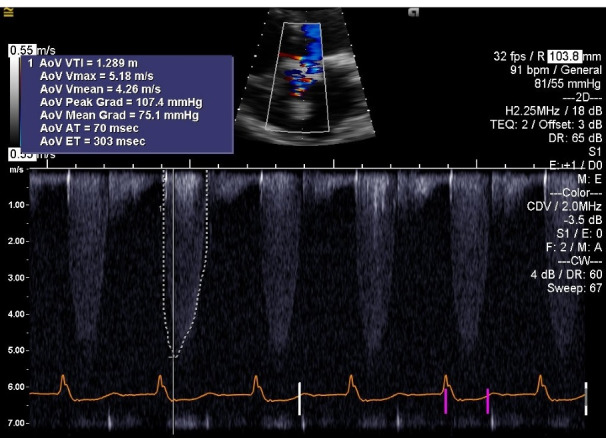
His acute decompensated heart failure was believed to be secondary to structural bio-prosthetic valve degeneration with associated critical stenosis and moderate regurgitation. Because of unstable hemodynamics, multiple comorbidities, high logistic Euro- score II of 67.0% and Society of Thoracic Surgery Risk (STS) score of 46%, the patient was deemed to be a poor surgical candidate for re-do surgical intervention. Therefore, he underwent immediate and successful TAVR with a ViV procedure with a 29 mm Medtronic Evout –R, using transfemoral approach with conscious sedation without complications. Follow up echocardiogram (Figure 2) one week post-procedure revealed prosthetic AV peak gradient of 64.3 mm Hg, mean gradient of 34.5 mm Hg, peak velocity of 4.01 m/s, and LVEF of 60%. The patient’s symptoms improved remarkably and he was discharged from the hospital after an extended stay of around 3 weeks. The patient had past medical history of CAD and was already on daily oral aspirin without any indication for concurrent dual antiplatelet therapy or any indication for anticoagulation. Another follow up echocardiogram at 5 months interval (Figure 3) revealed prosthetic AV peak gradient of 39.8 mm Hg, mean gradient of 23.0 mm Hg, and peak velocity of 3.15 m/s.
